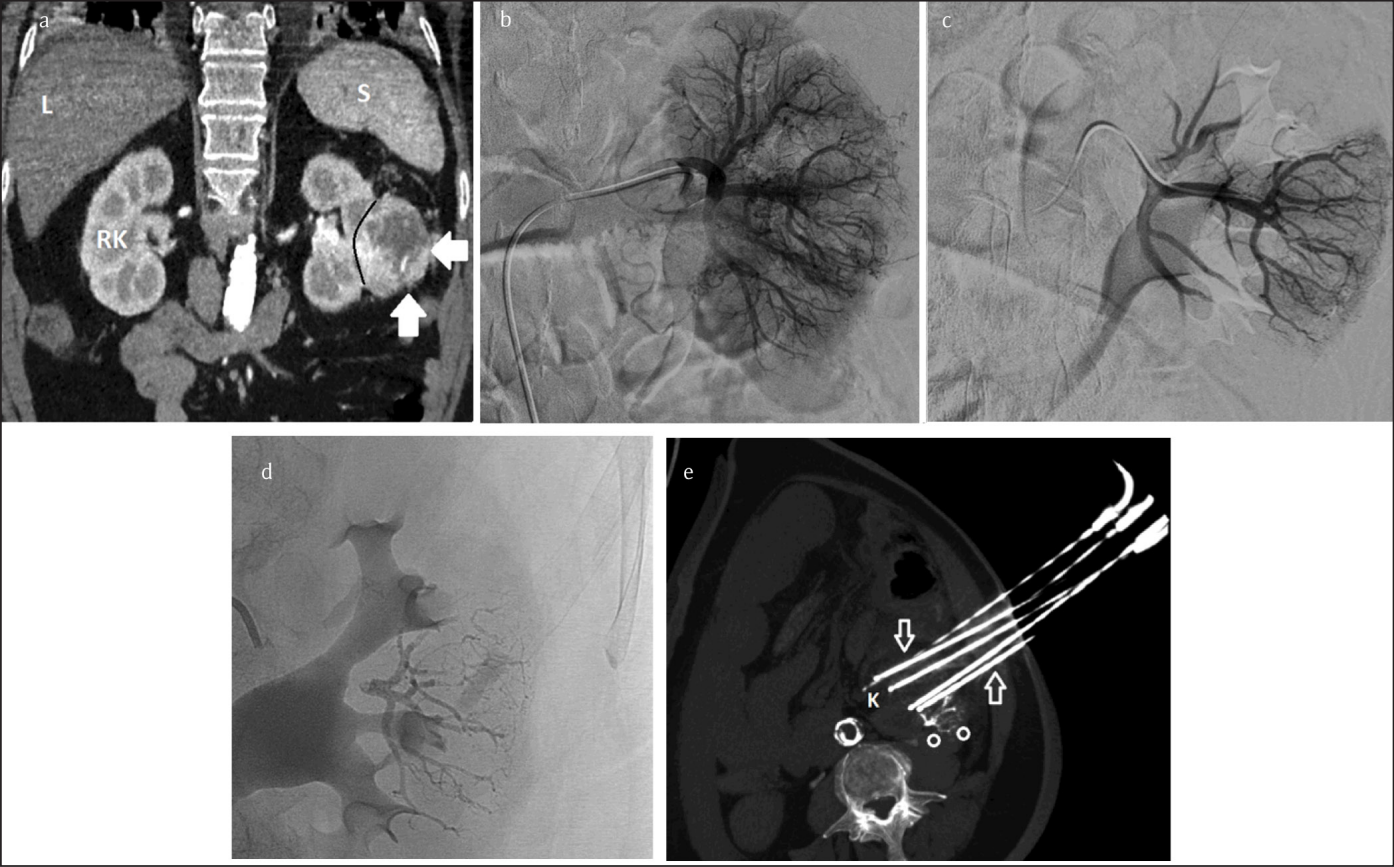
Interventional management of a renal mass in an inoperable patient, combined approach: transarterial preoperative embolization is performed through femoral access (b) using selective catheterization (c) and injection of glue (d), thus obtaining devascularization before percutaneous ablation. (e) CT-guided cryotherapy; combination of the percutaneous ablative therapies and the immune-modulating drugs in the so-called “Interventional Immunooncology” becomes an unexplored field of application with a tremendous potential to investigate in the next future.
